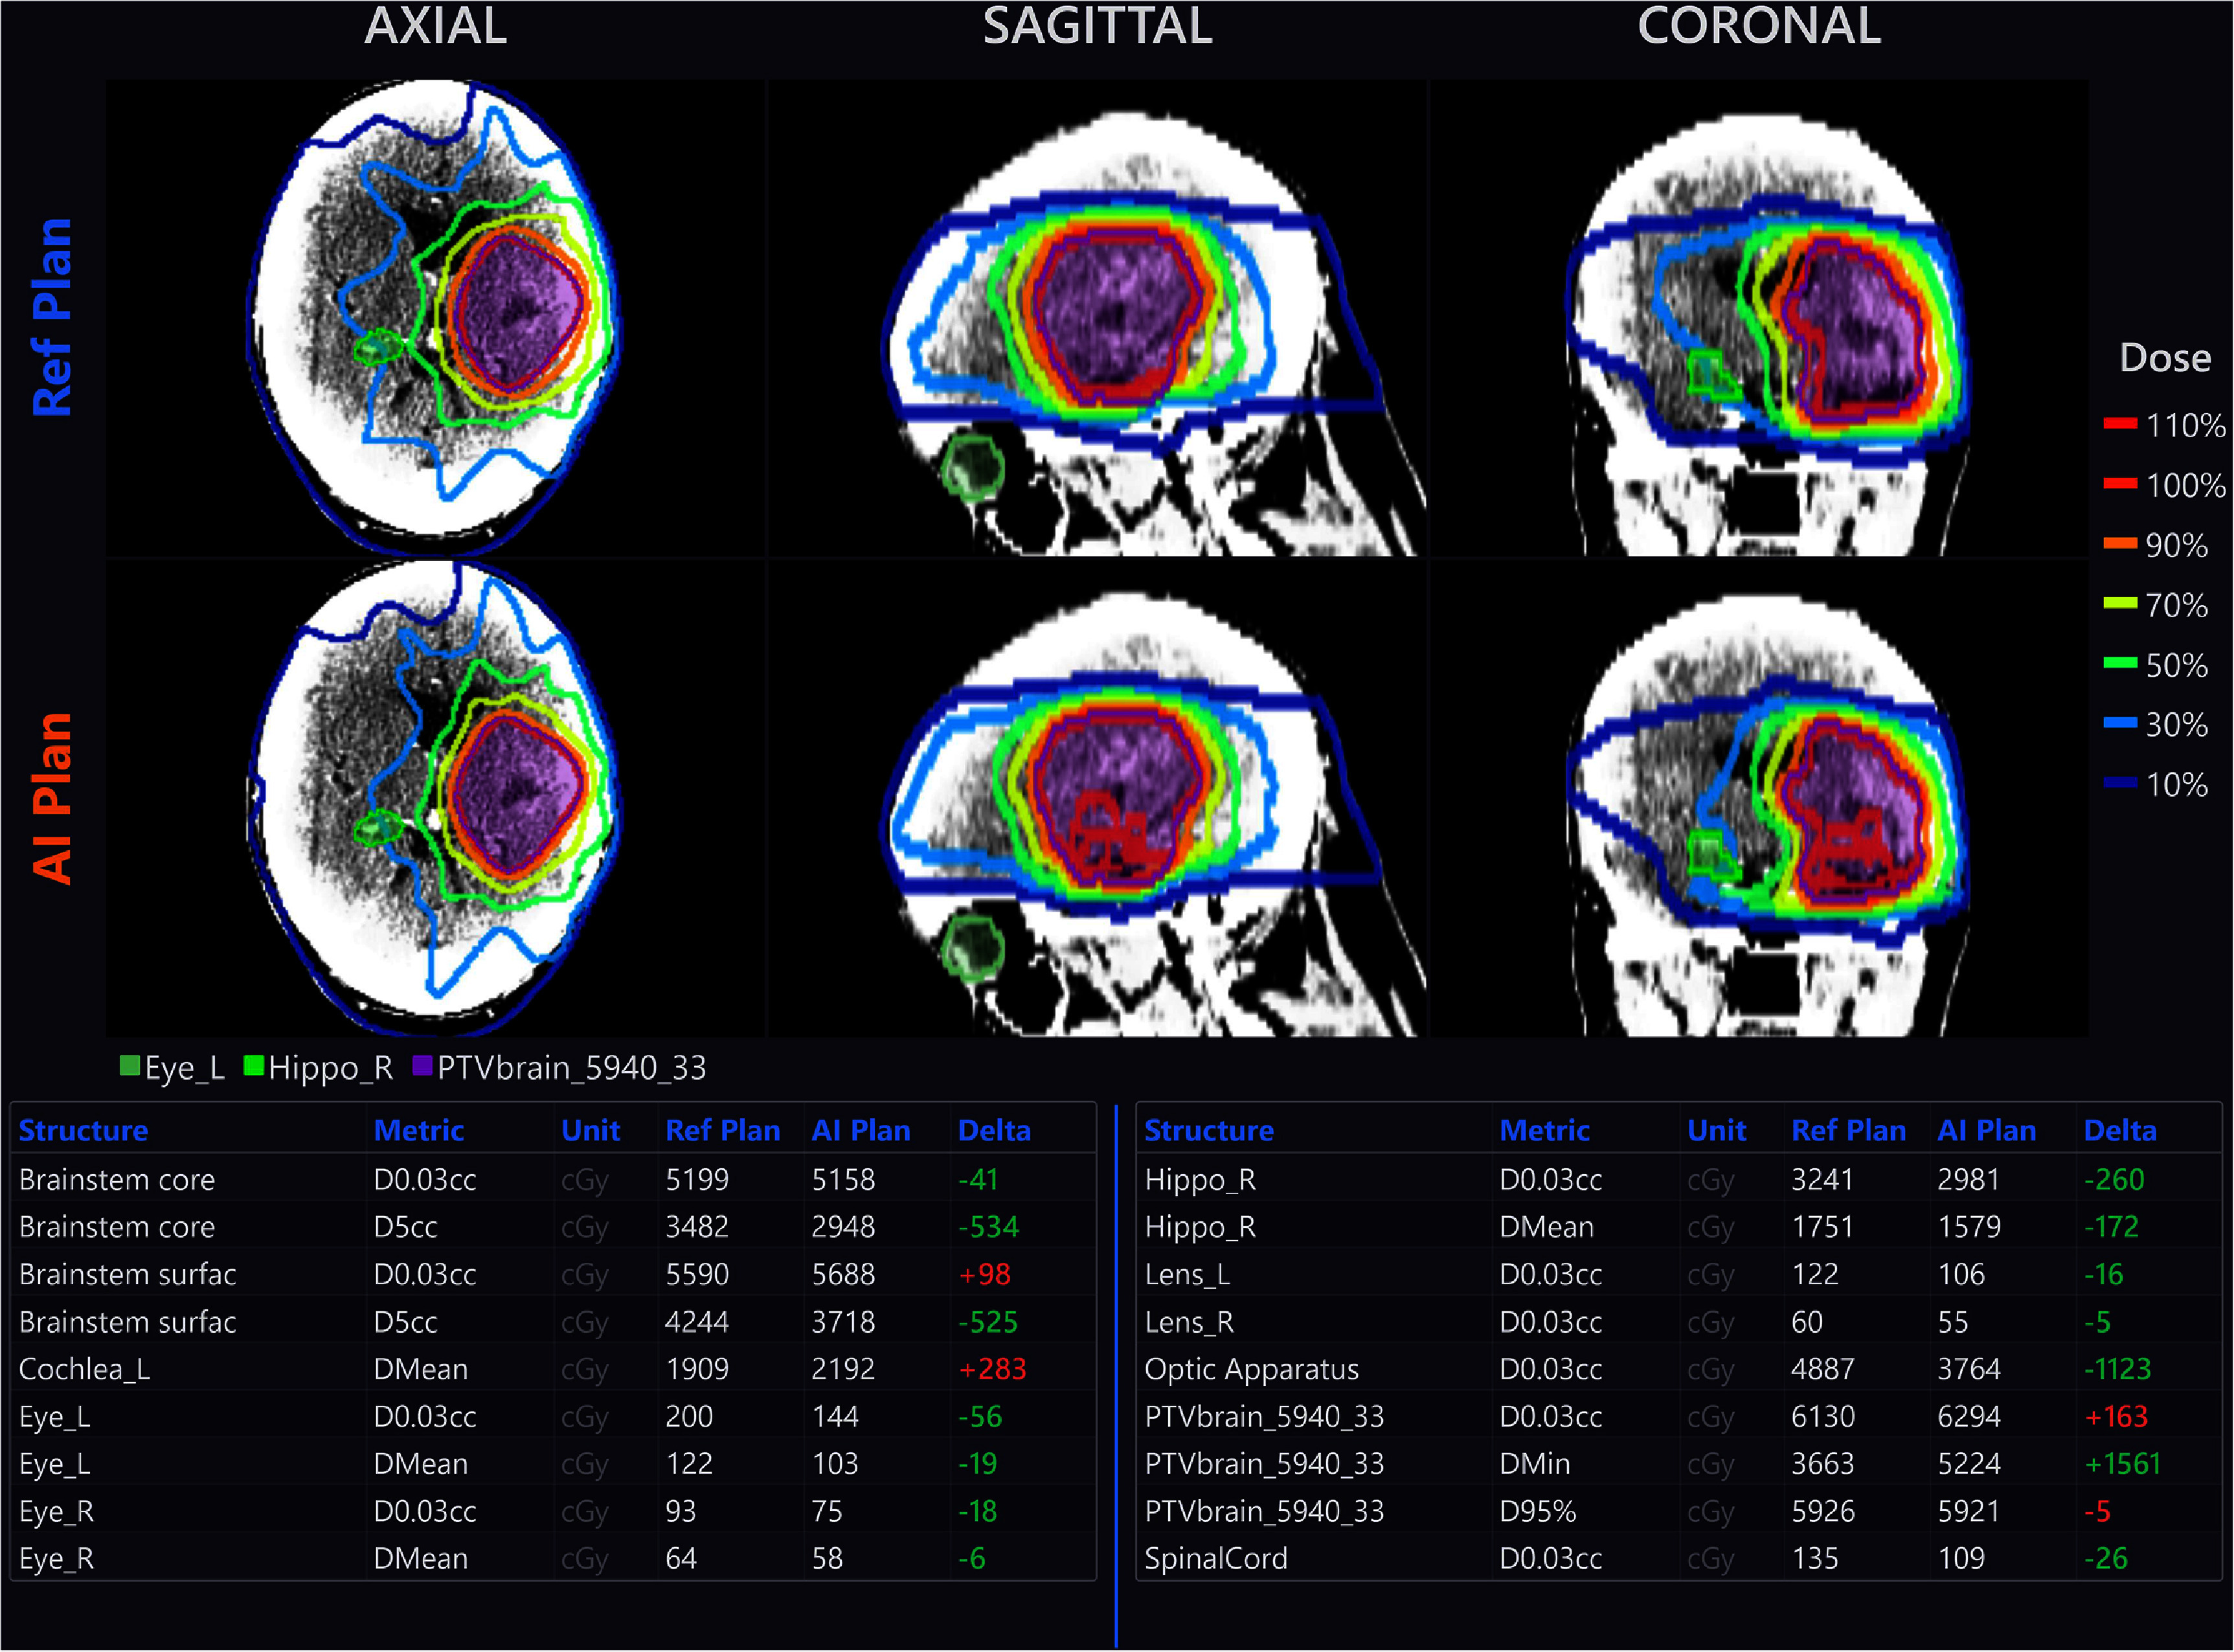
A brain case that receives 59.4 Gy in 33 fractions. Top row: clinical reference plan. Bottom row: artificial intelligence (AI) plan. Dose is overlaid on axial computed tomography (CT) images with the planning target volume (PTV) (purple), left eye (green), and right hippocampus (light green) contours displayed. Below the images is a dose-volume histogram (DVH) metric comparison between the two plans. The AI plan substantially improved the minimum dose to the PTV while also substantially reducing dose to the optics.
